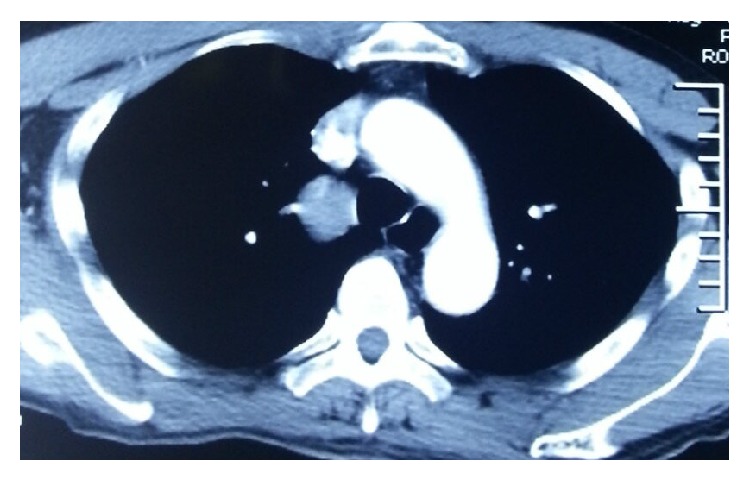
Contrast computed tomography confirmed a slowly enhancing mass with a smooth margin adjacent to the right side of the trachea in the pathway of the azygos arch.
